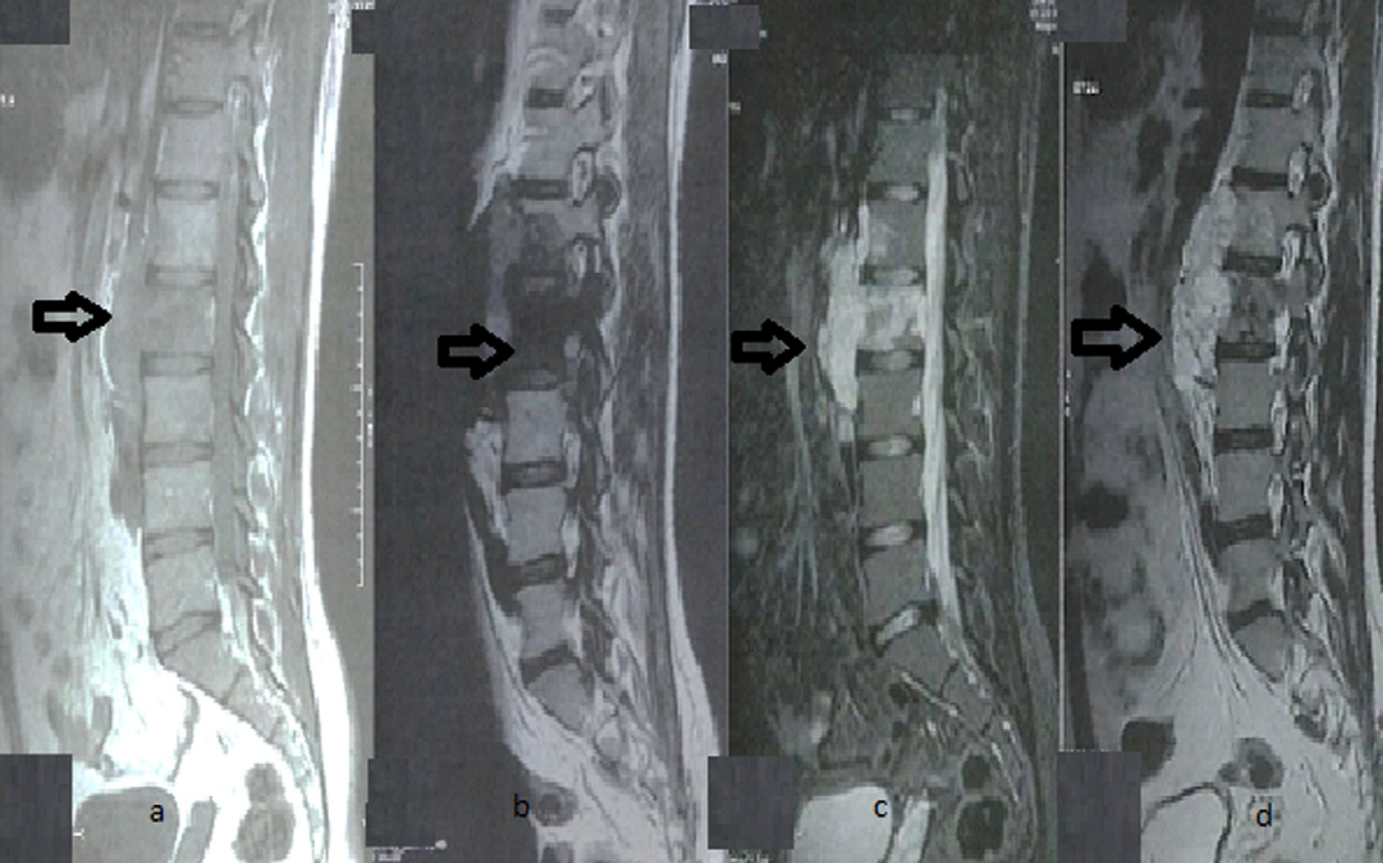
T1 (A , B) T2 (C, D)-weighted sagittal MRI of lumbar spine showing bone-based malignancy from L1 to L3.
